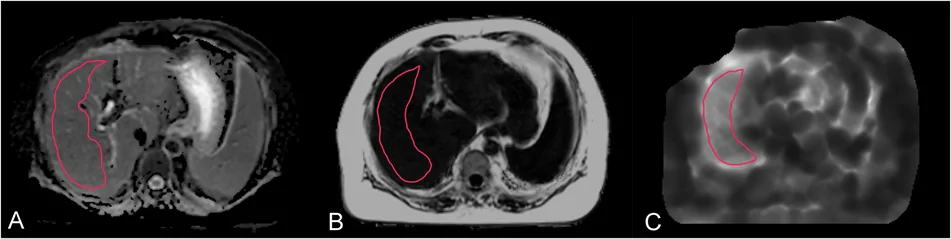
Representative region of interest (ROI) placement on multiparametric MRI maps in a patient with metabolic dysfunction-associated steatotic liver disease (MASLD). A Apparent diffusion coefficient (ADC) map, (B) proton density fat fraction (PDFF) map, and (C) magnetic resonance elastography (MRE) elastogram
